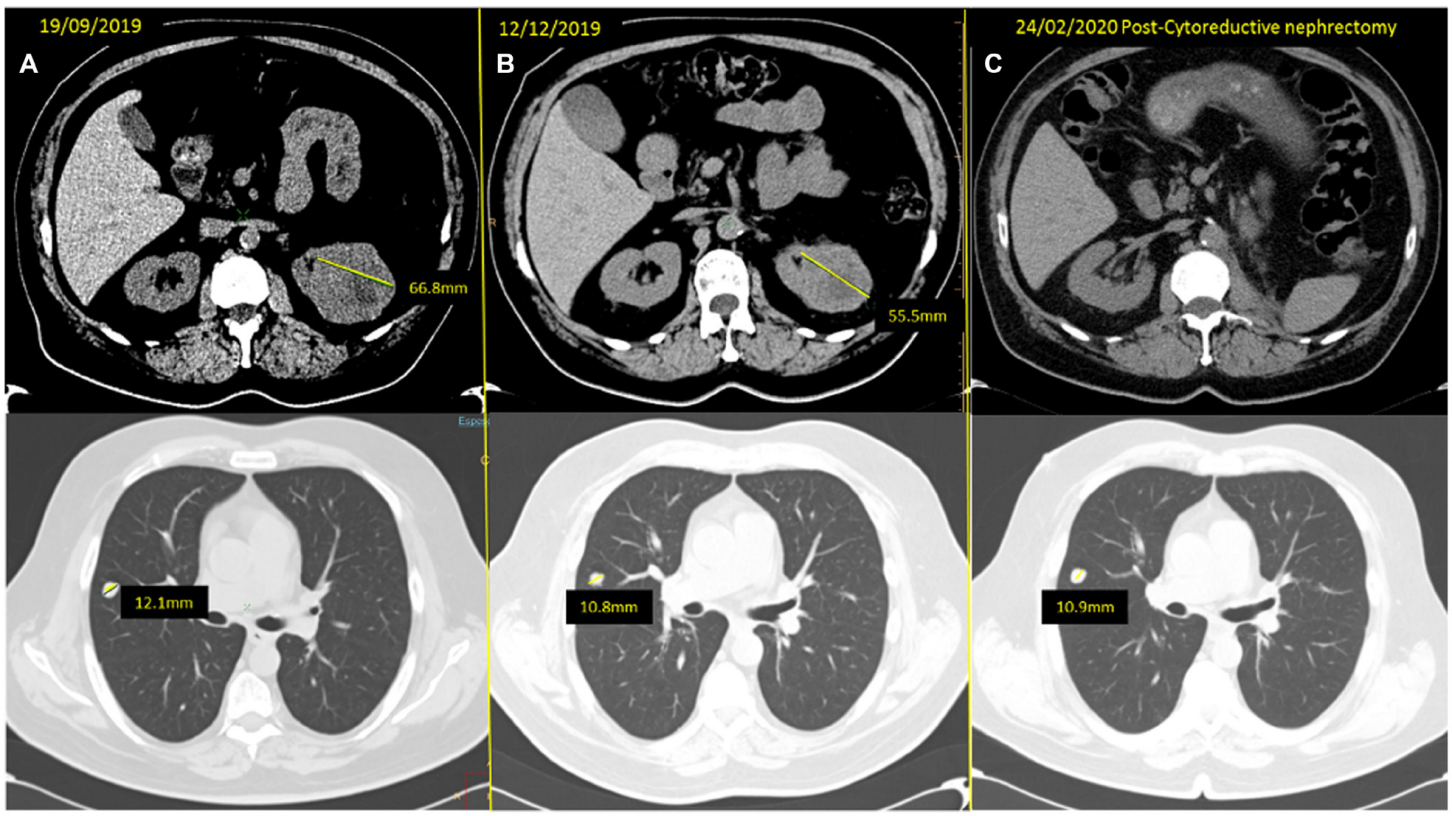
(A) baseline CT scan and (B) response obtained after three months of neoadjuvant cabozantinib, with a reduction of the left kidney mass from 66.8 to 55.5 mm and of the SRL nodule from 12.1 to 10.8 mm. (C) Last reassessment CT scan, after CN, showing only stability of the remaining solitary lung metastases which is pending surgery.
